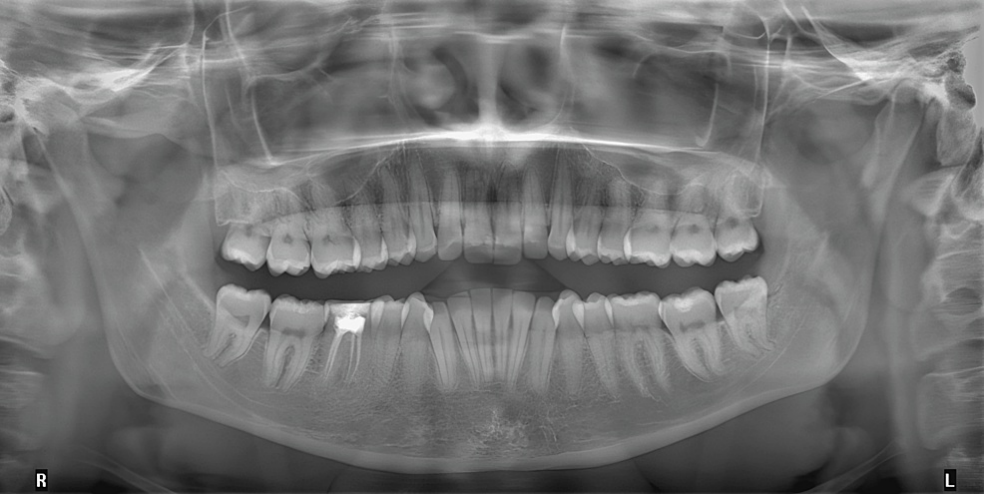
Pre-treatment orthopantomogram
